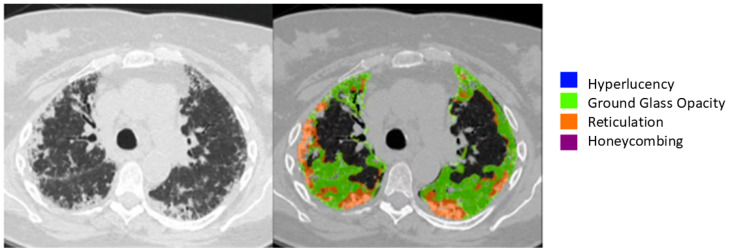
Side-by-side comparison of a representative axial HRCT slice (left) and its corresponding AI-processed segmentation (right) using the Imbio Lung Texture Analysis (LTA) platform. The algorithm classifies lung parenchyma into four radiological patterns—ground-glass opacity (yellow), reticulation (red), honeycombing (orange), and hyperlucency (blue)—as shown in the color-coded legend. Automated segmentation highlights areas of abnormality with precision and reproducibility, facilitating objective quantification of disease extent in CTD-associated ILD.
